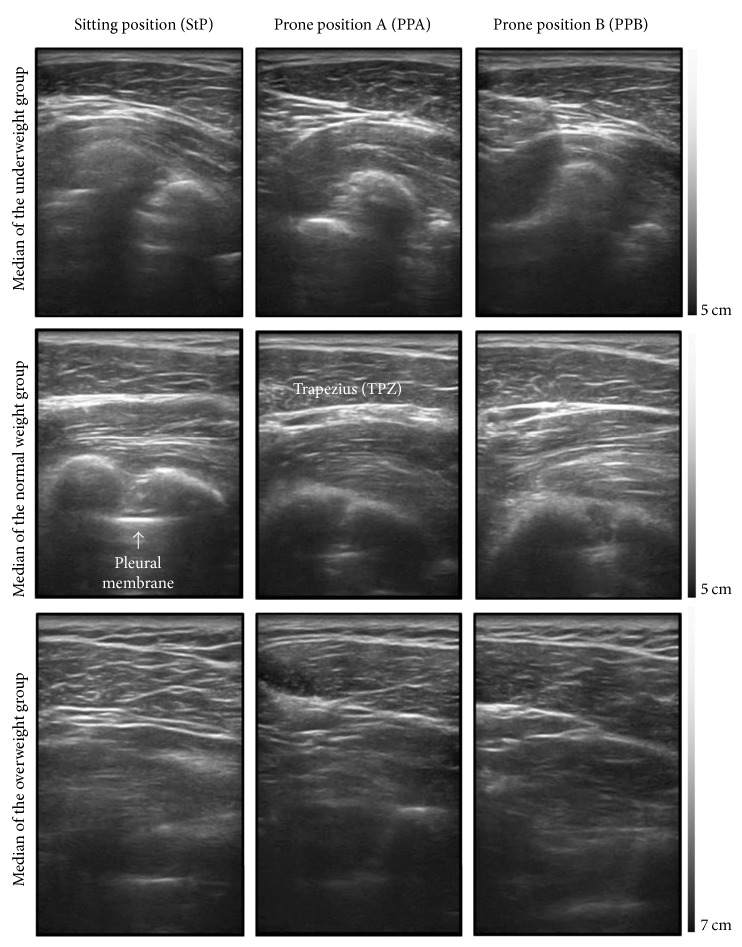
Ultrasound images of GB21 according to the body mass index (BMI) groups and positions.
